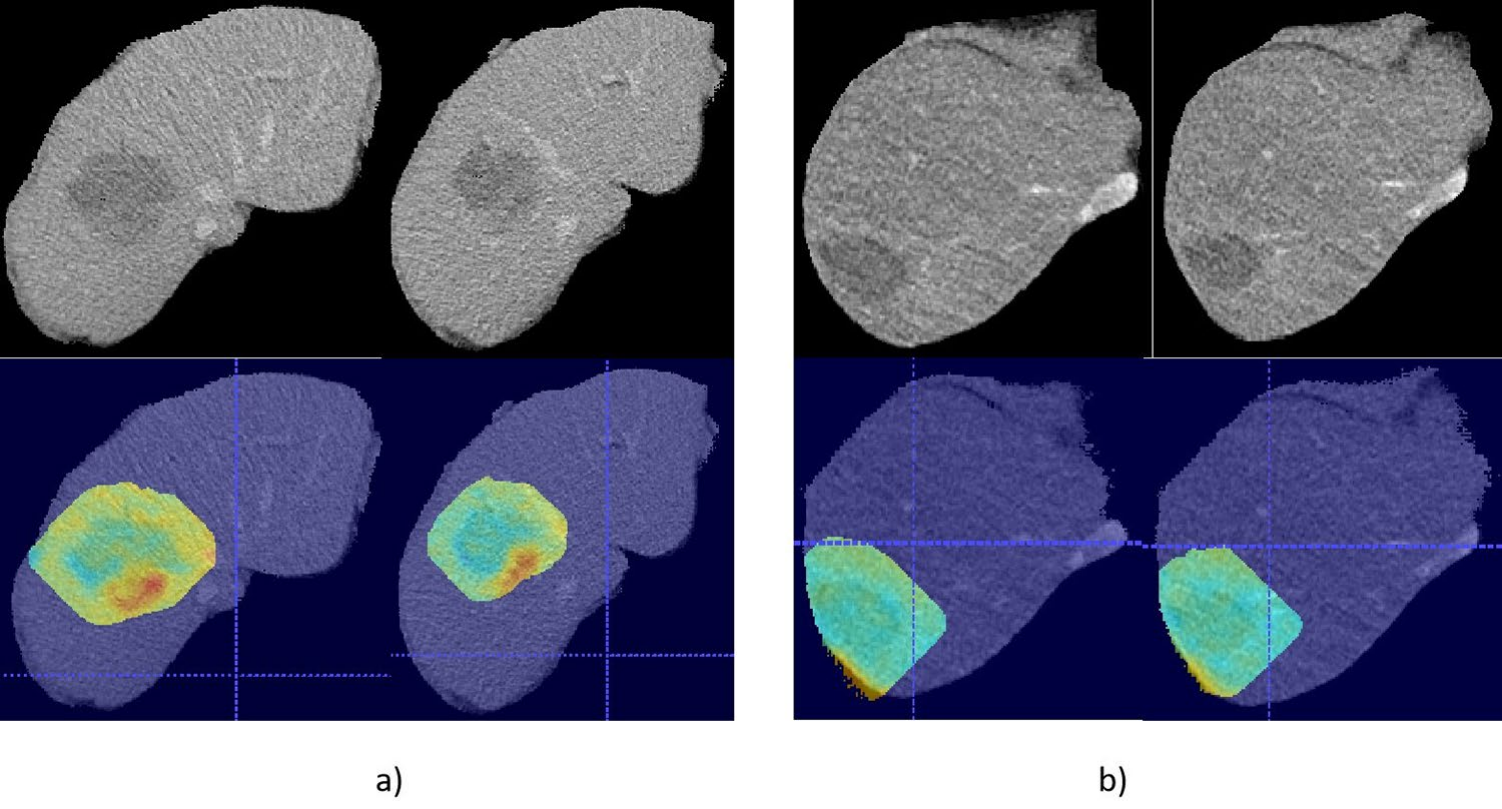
An example of diff-maps overlaid on a CT scan for two different scenarios: a The diff-maps for a patient with a post-ablative liver tumor recurrence, where the recurrent local tumor is highlighted with red color on the diff-maps. b The diff-maps for a patient without any post-ablative liver tumor recurrence, where no region is emphasized in red color on the diff-map
